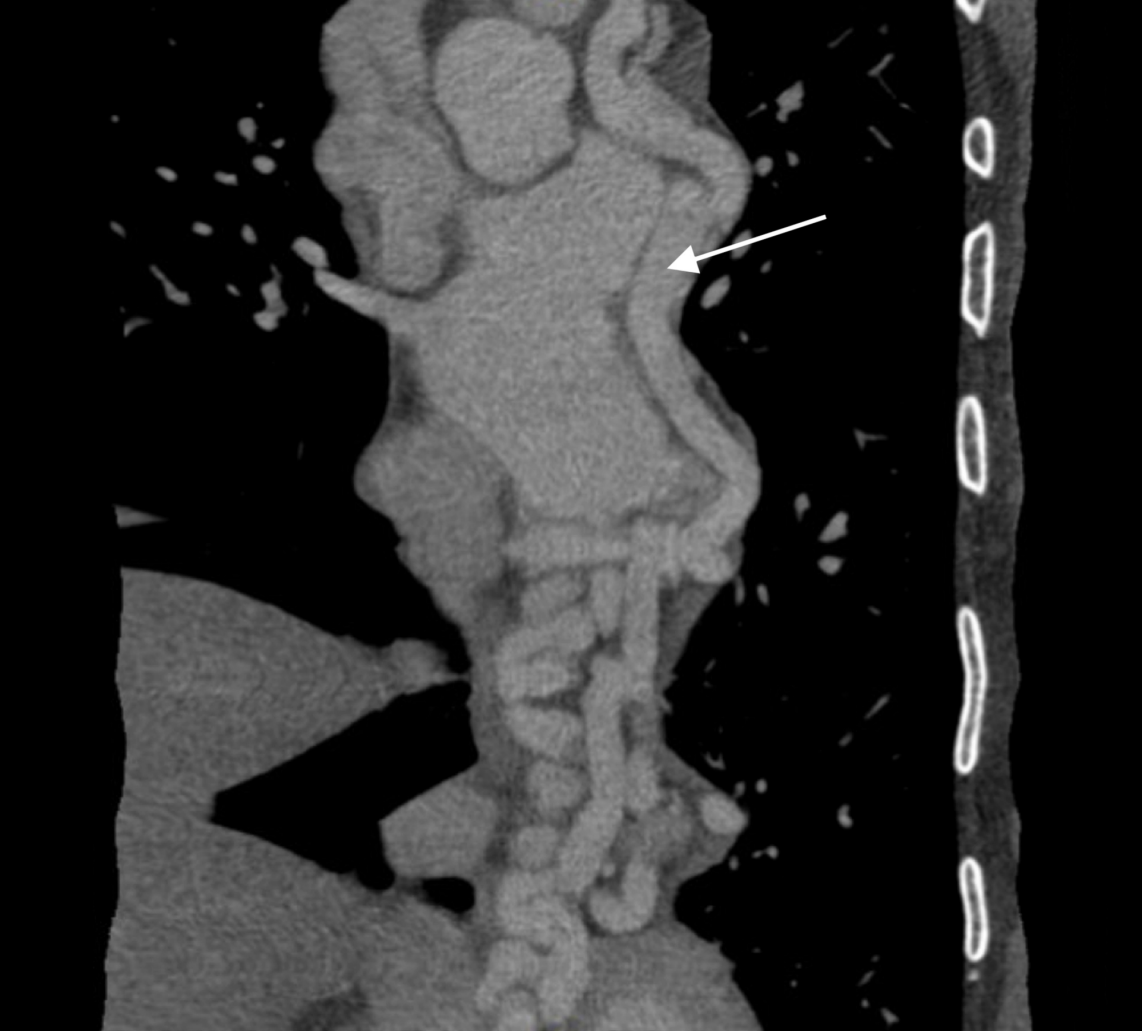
Coronary CT angiogram showing fistula extending from left circumflex artery (LCX) to coronary sinus (white arrow).
